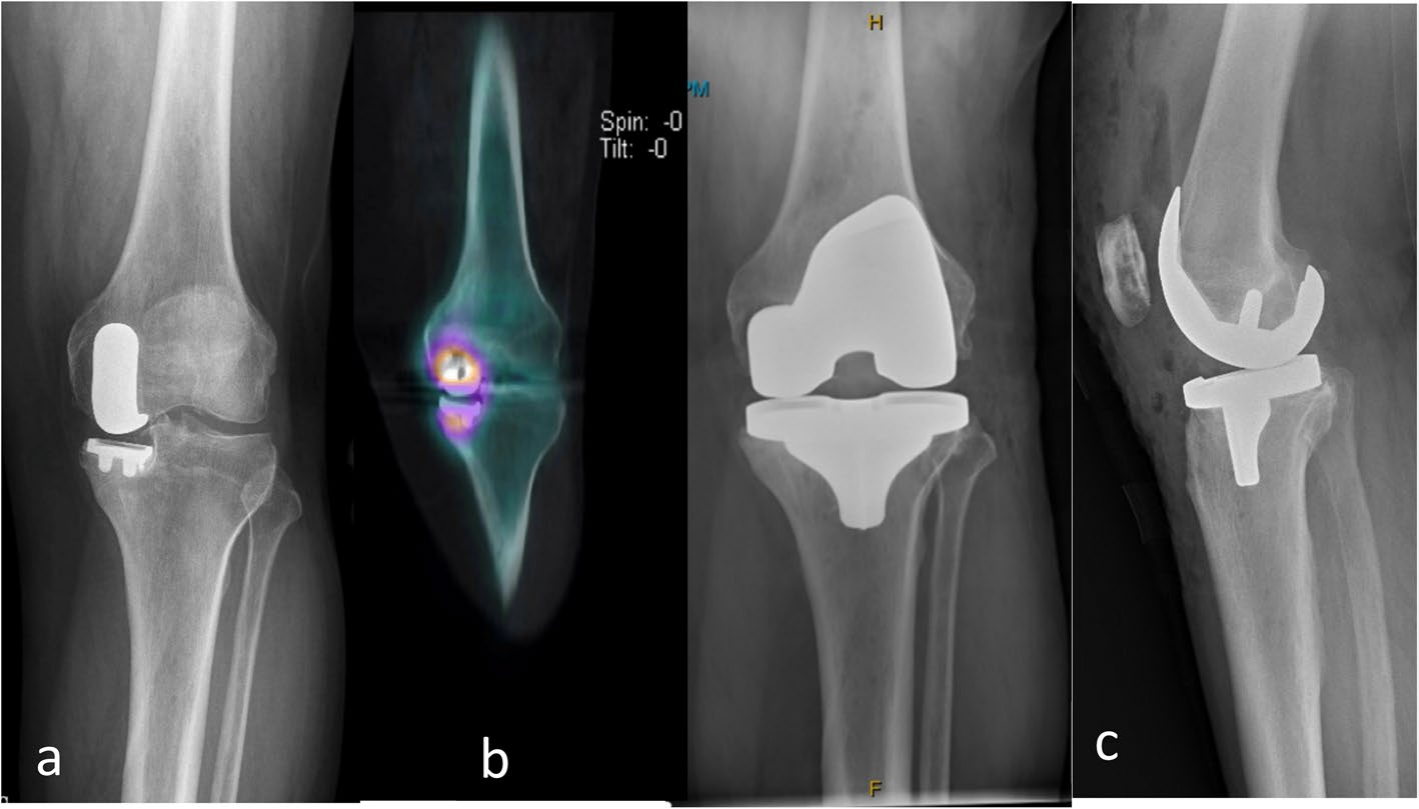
a Preoperative knee radiograph of the patient with the preoperative plan from Fig. 11; b Spect CT of the knee of the same patient showing loosening of the UKA; c Postoperative knee radiograph of the same patient
